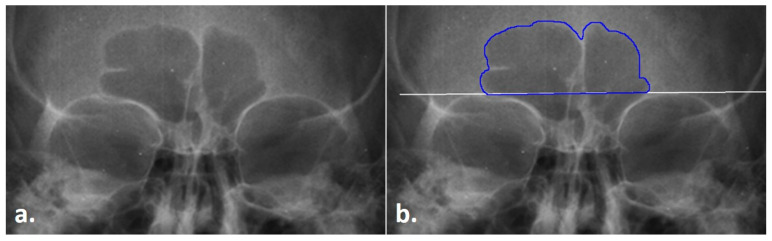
Radiograph of the frontal sinus; (a) untreated radiograph, (b) frontal sinus with the outline (blue) and supraorbital line (white).
